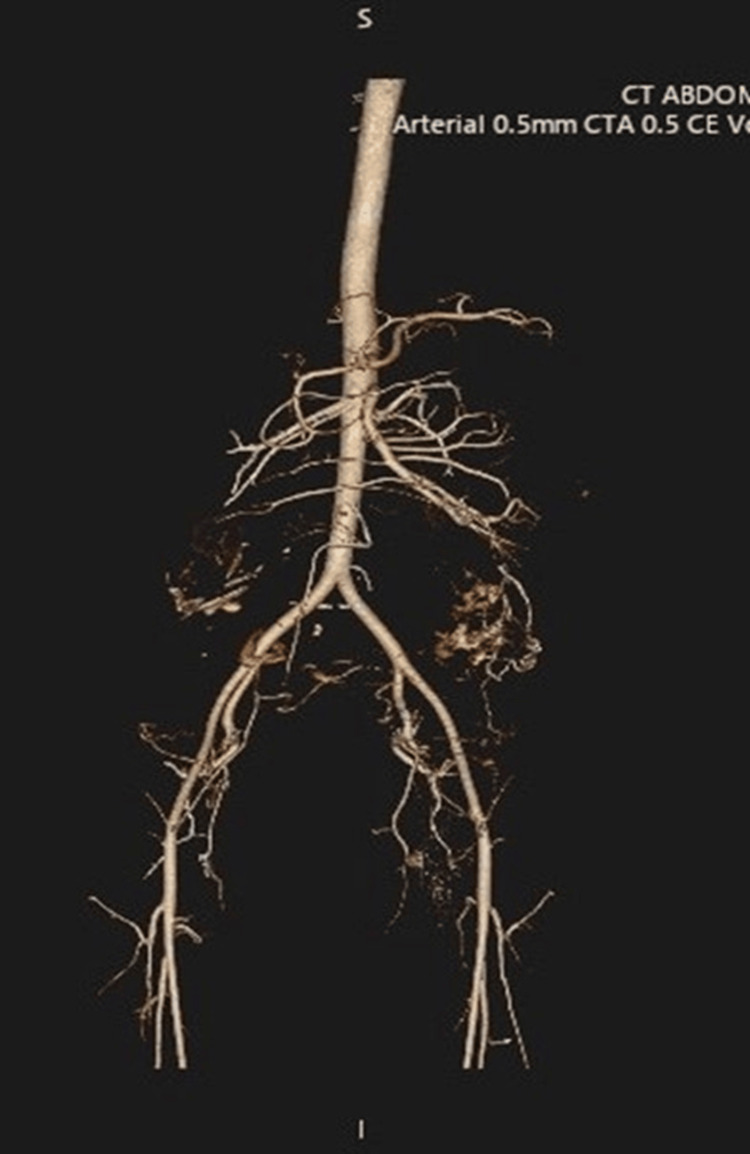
CT angiogram postoperatively shows major pelvic and abdominal vasculature feeding the placental bed
